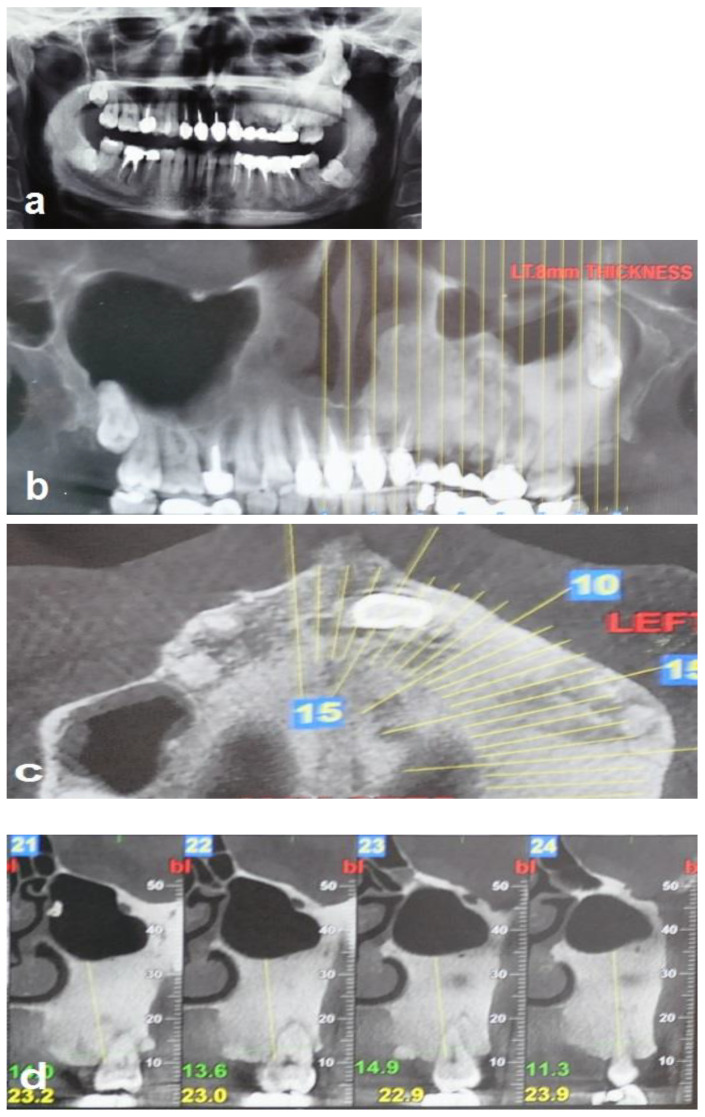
Radiographic views demonstrate that the FD lesion involves the left zygomaticomaxillary complex. (a,b) Panoramic radiograph and coronal CBCT show a hyperdense lesion in the left hemi-maxilla with displacement of the left maxillary sinus and the wisdom tooth, respectively. (c) Axial CBCT shows the hyperdense lesion involving the left maxilla, and (d) sagittal (cross-sectional) CBCT shows bone sclerosis and the displacement of the left maxillary sinus.
